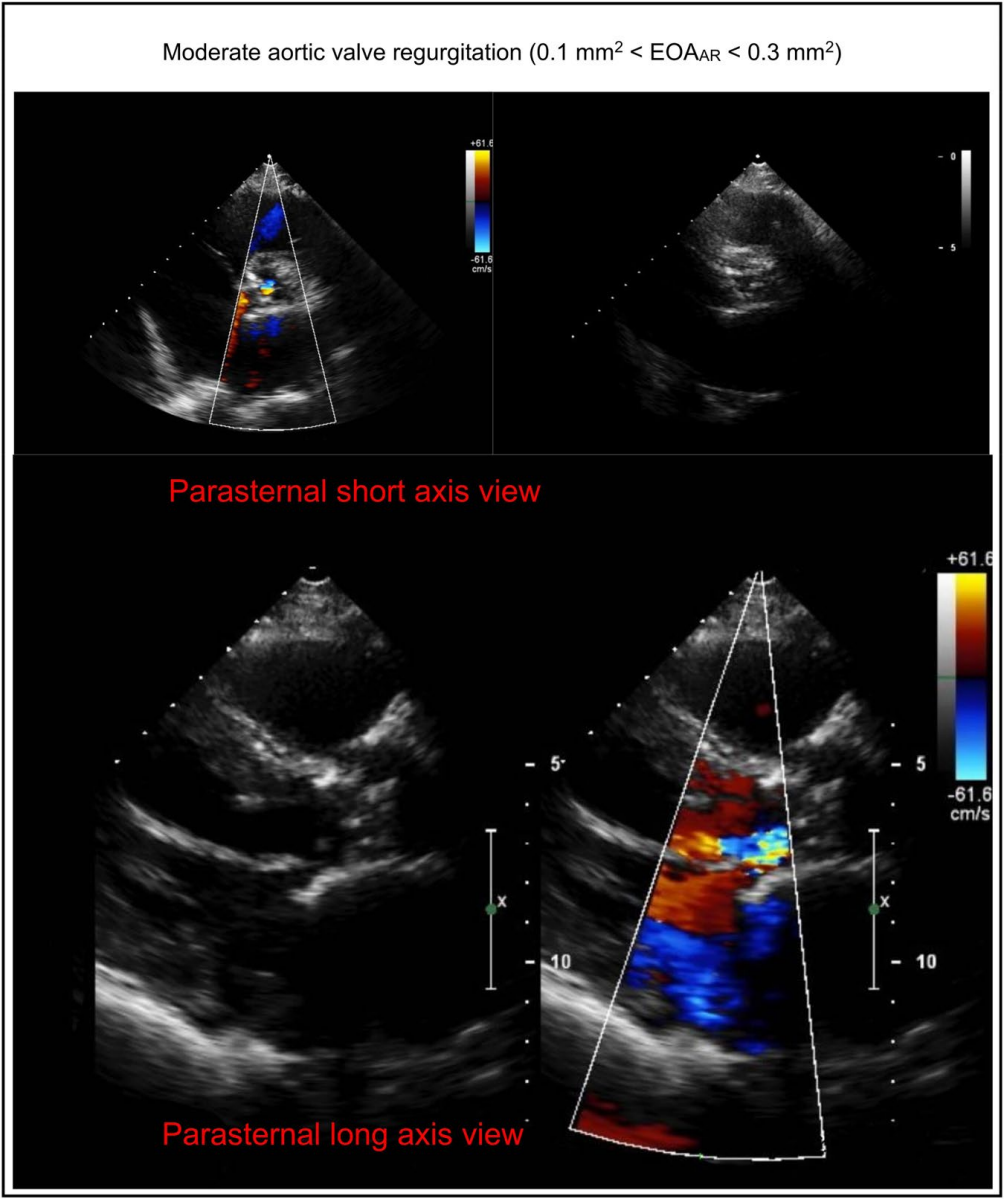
Doppler echocardiography investigation for aortic valve regurgitation. To evaluate aortic valve regurgitation severity, aortic valve color Doppler images are used in both long axis, and short axis views. This image is an example of moderate to severe aortic valve regurgitation in a patient with AS who received TAVR (0.2 mm^2^ < EOA_AR_ < 0.3 mm^2^).
